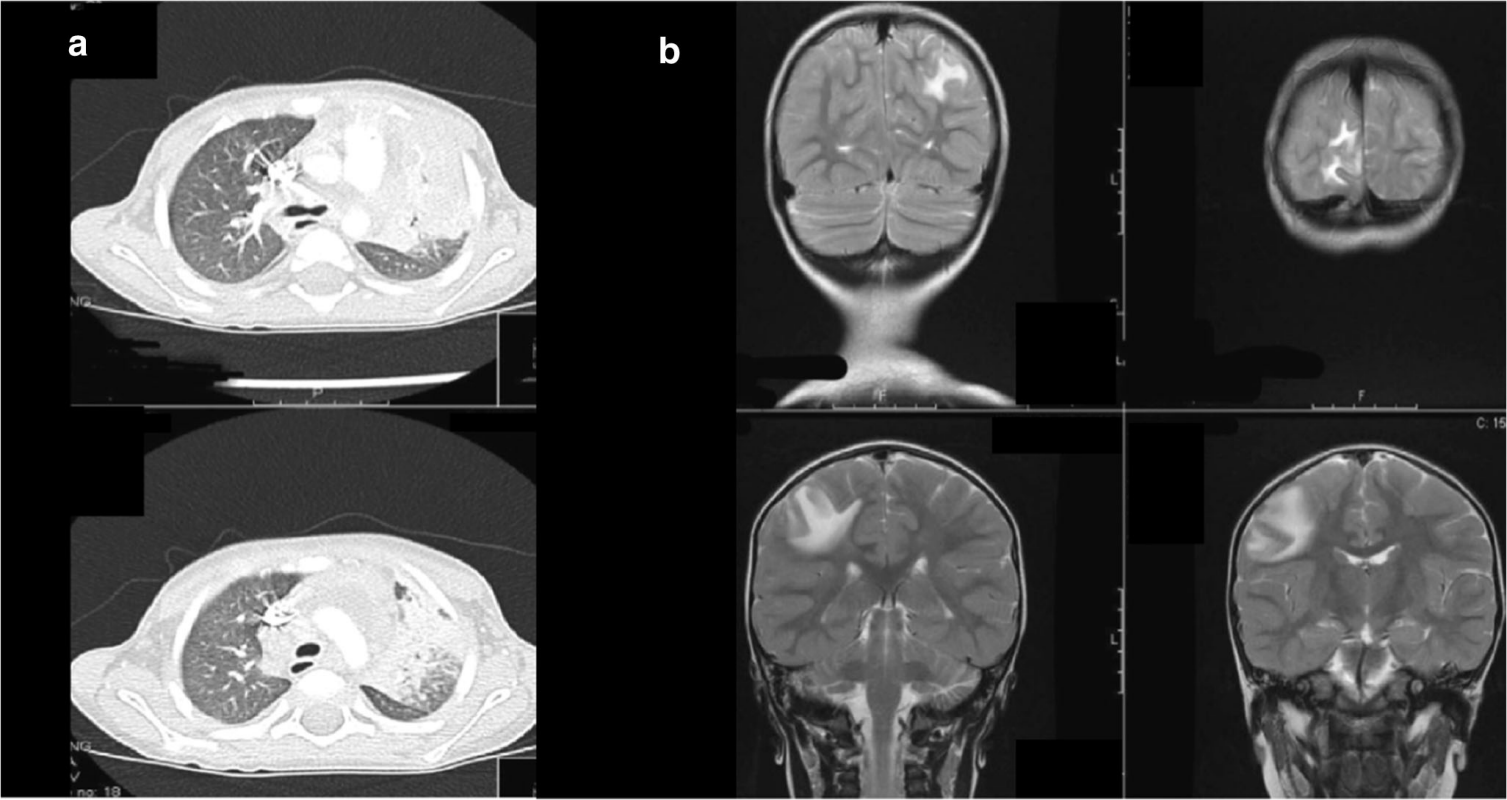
Chest CT scan of patient with mycobacterial infection and brain MRI lesions. a Chest CT scan: parenchymal consolidation of left upper lobe and diffuse lymphadenopathies. b Brain MRI: multiple hypodense cortical and subcortical lesions in the right frontoparietal and in less extent in right parietal and occipital lobes with perilesional edema
